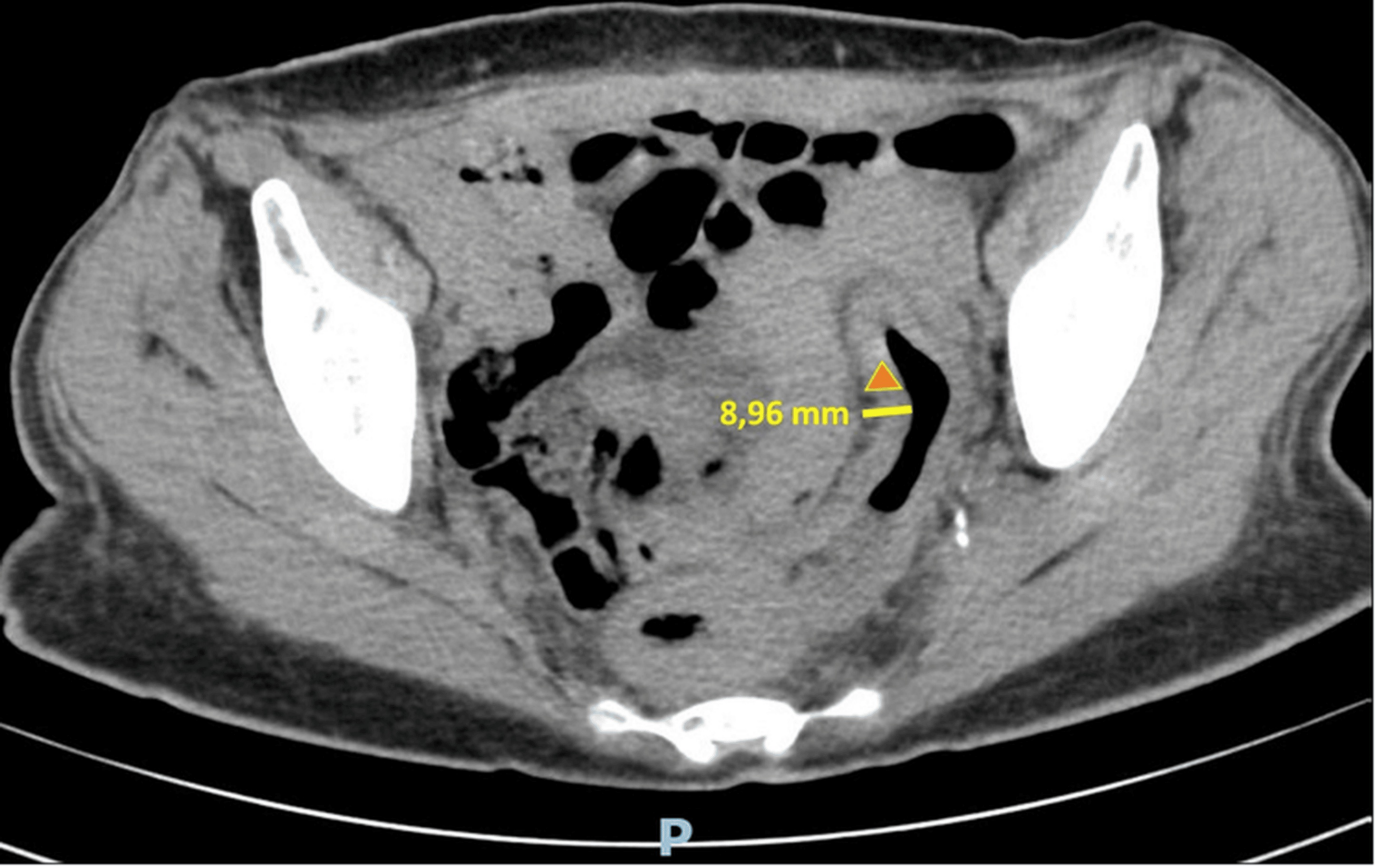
Pelvic CT (image 1 of 2) Axial CT image showing diffuse thickening of the colon (▲), compatible with active inflammation.
